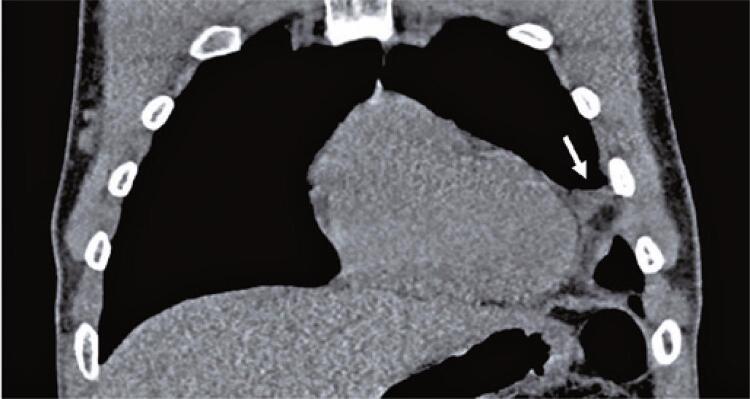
Computed tomography of the chest on coronal view evidencing a rounded lesion (arrow) with fat attenuation and variable degrees of densification of the adipose planes
